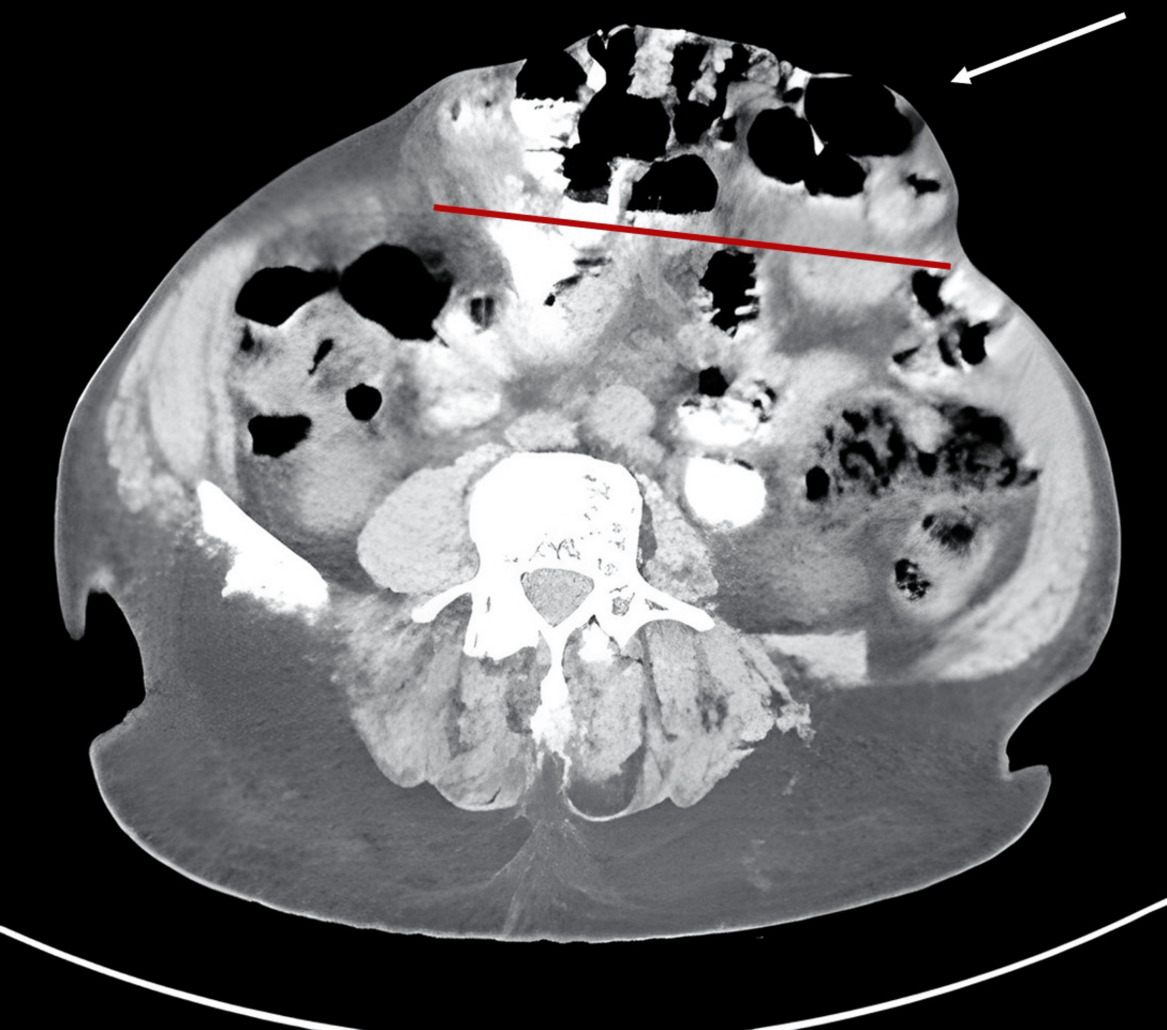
Abdominal CT scan showing the incisional hernia classified as M2W3
